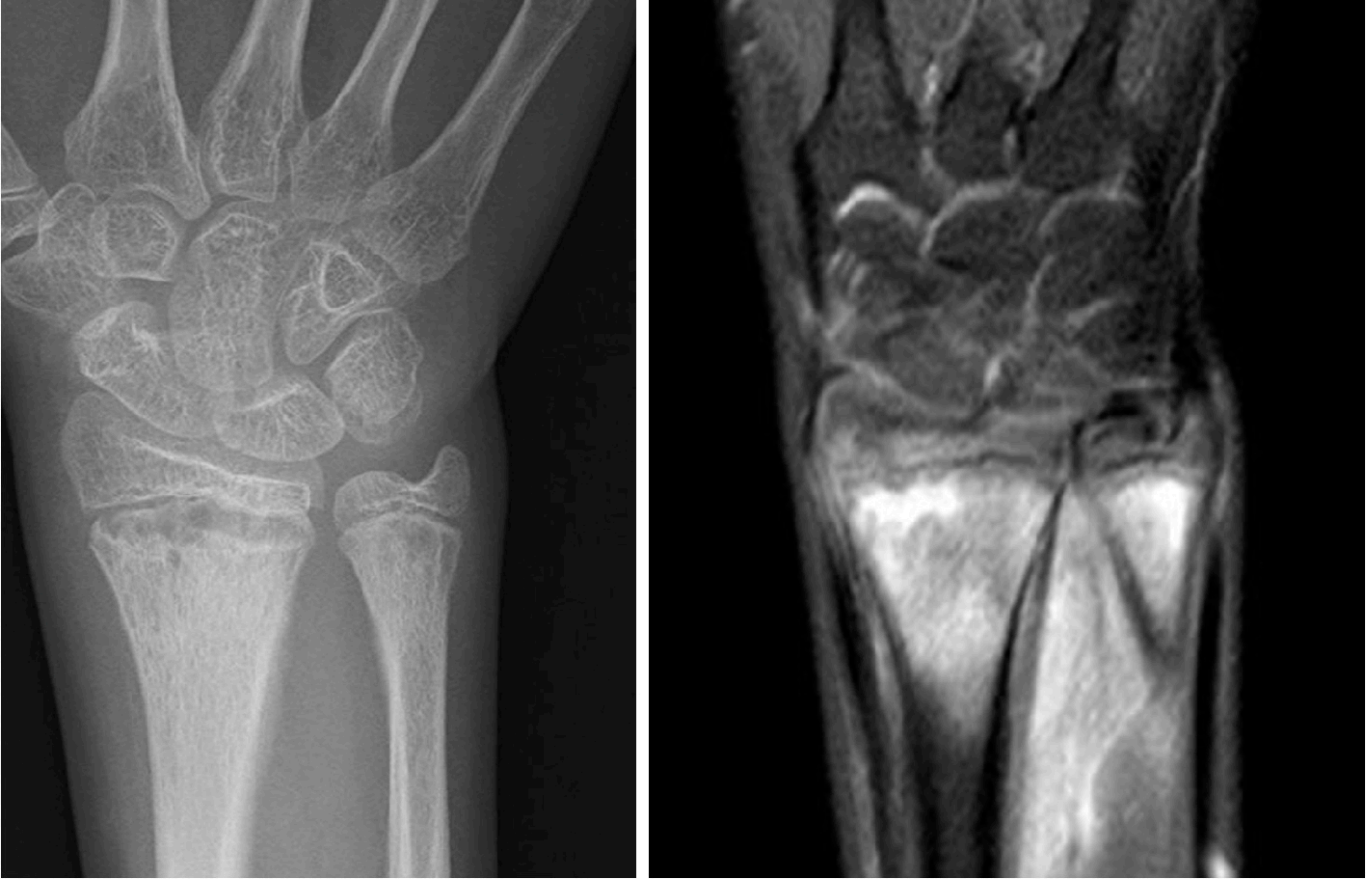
Radiograph and MRI of the heel performed on the initial visit. MRI with STIR sequences demonstrated bone marrow edema. MRI: magnetic resonance imaging; STIR: short tau inversion recovery.
